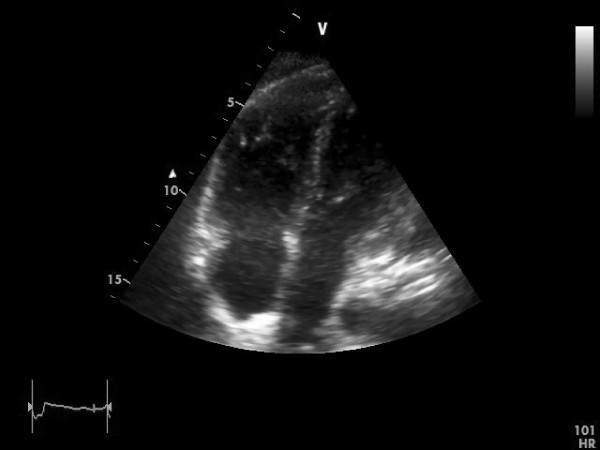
TTE on day 2 after thrombolytic therapy. Apical 4-chamber view shows complete disappearance of the right heart thrombus.
